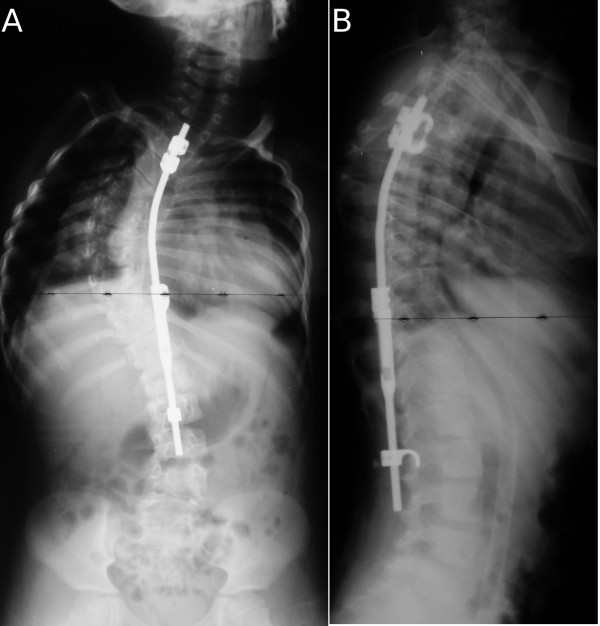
(A, B), Case 4: Postoperative radiographs, anteroposterior (A) and laterolateral (B) view.
